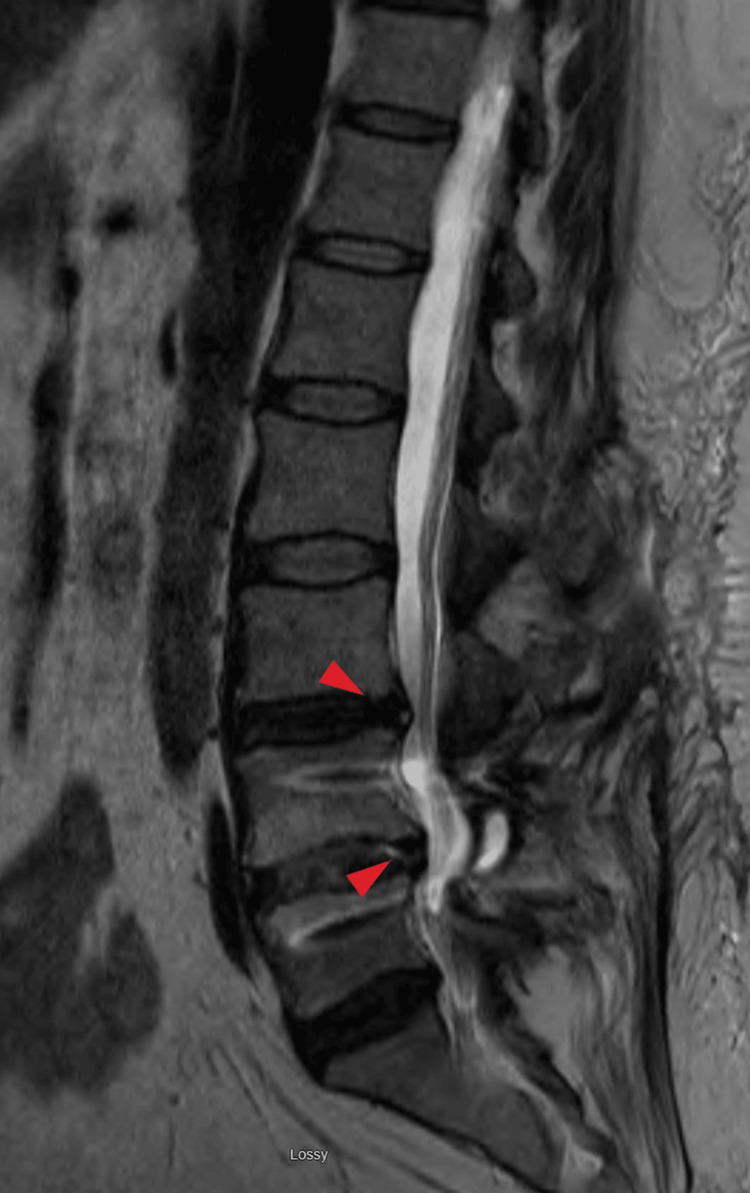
MRI of the lumbar spine shows interval postoperative changes at the L4-5 level, and degenerative changes at L3-4 causing mild central canal compromise (Age: 55 years).
